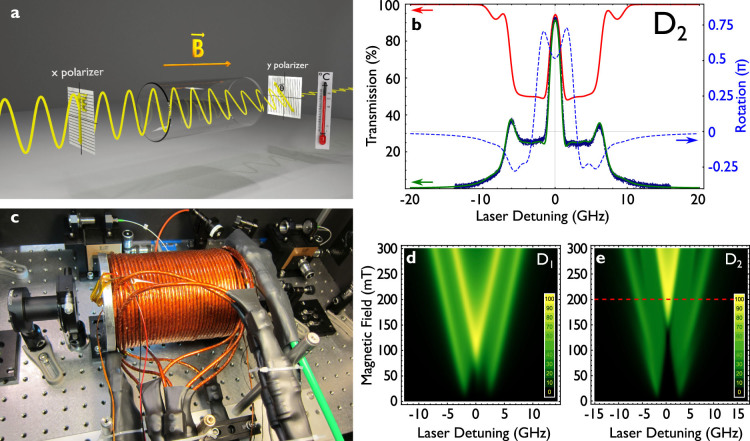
(a) general scheme of the discussed Faraday filter: an atomic vapour cell is placed between two crossed linear polarizers (wire-grid type shown). A magnetic field (B) leads to a Zeeman split of the involved levels, which then act differently on both circular polarization components. An overall turn of the polarization results in the transmission through the second polarizer (b) calculated (green) and measured (blue, dots) transmission spectrum of the D_2_ transition of sodium for 153°C and 0.2 T. Optical rotation (blue, dashed), Doppler spectrum (red, OD approx. 5). (c) experimental setup of Na-FADOF (d) density plot of the transmission for a temperature of 153°C for various magnetic fields, Na-D_1_transition. (e) same for the D_2_ transition. The red dashed line corresponds to the transmission spectrum in (b).
